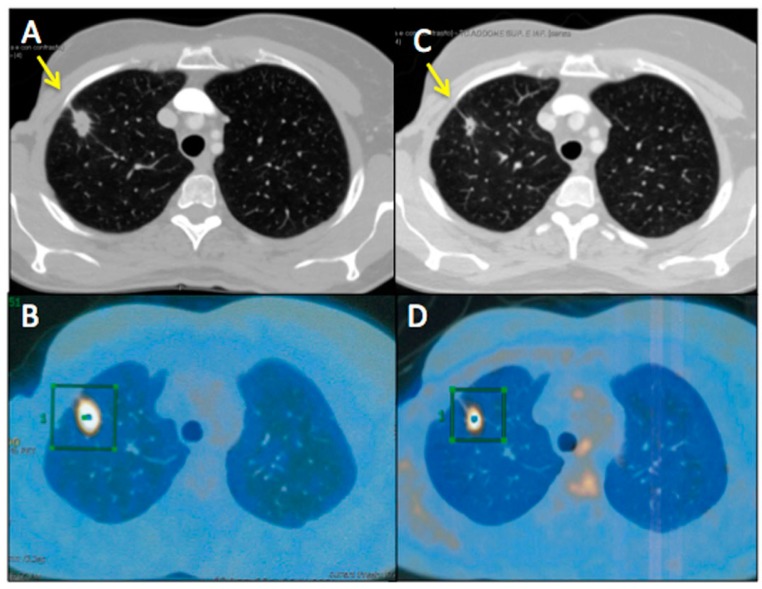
A 55-year-old female affected by breast cancer with lung metastasis, subjected to 12 cycles of Bevacizumab-based chemotherapy and 24 hyperthermia sessions on the thorax, as first-line. Baseline Multidetector Contrast Enhancement Computed Tomography (MDCT) (A) showed metastasis in the superior right lobe of the lung (diameter: 17 mm × 12 mm, yellow arrow), confirmed by the increased ^18^F-Fluorodeossiglucose (^18^F-FDG) uptake on Positron Emission Tomography/CT (PET/CT) images in the same sites (green square, B). Timepoint-2 MDCT (C) evaluation demonstrated size decrease of lung metastasis (diameter 14 mm × 7 mm, yellow arrow) with ^18^F-FDG uptake decrease on PET/CT images (green square, D). According to mRECIST, patient was classified as PR. Ca15.3 and CEA values evaluated at baseline were 830 UI/mL and 135 ng/mL, respectively; while Ca15.3 and CEA values evaluated at timepoint-2 were 115 UI/mL and 40 ng/mL, respectively. Side effects reported were limited to asthenia and peripheral sensory neuropathy.
